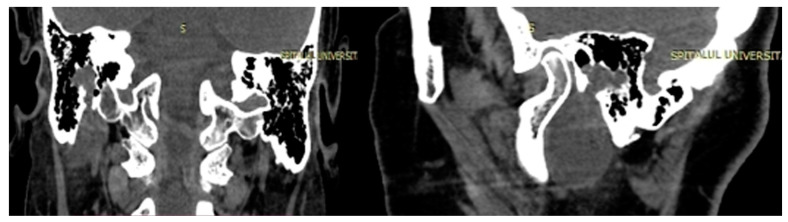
Temporal bone CT showing facial nerve schwannoma developed at the level of the stylomastoid foramen with minimal extension into the mastoid segment of the facial nerve (coronal and sagittal sections).
